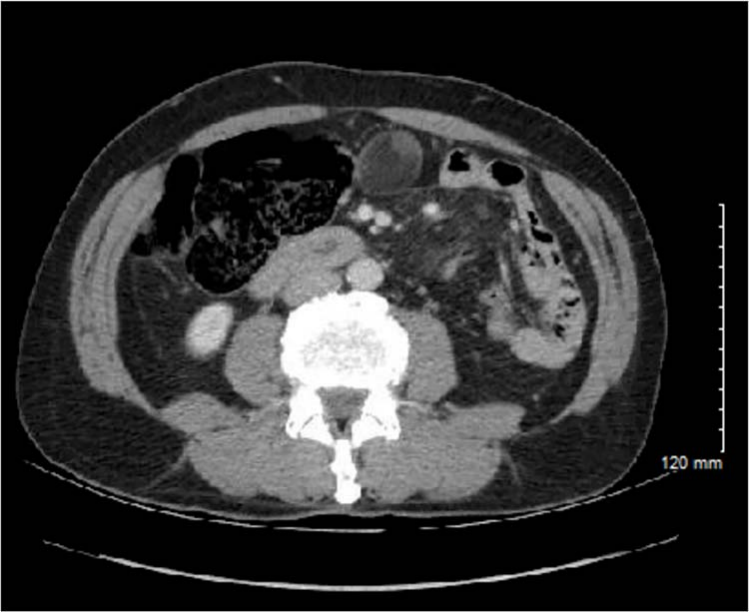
Initial CT imaging showing an extraluminal mass, measuring 3.6 × 3.0 × 3.9 cm with mural soft tissue nodularity within the midline anterior abdomen.
